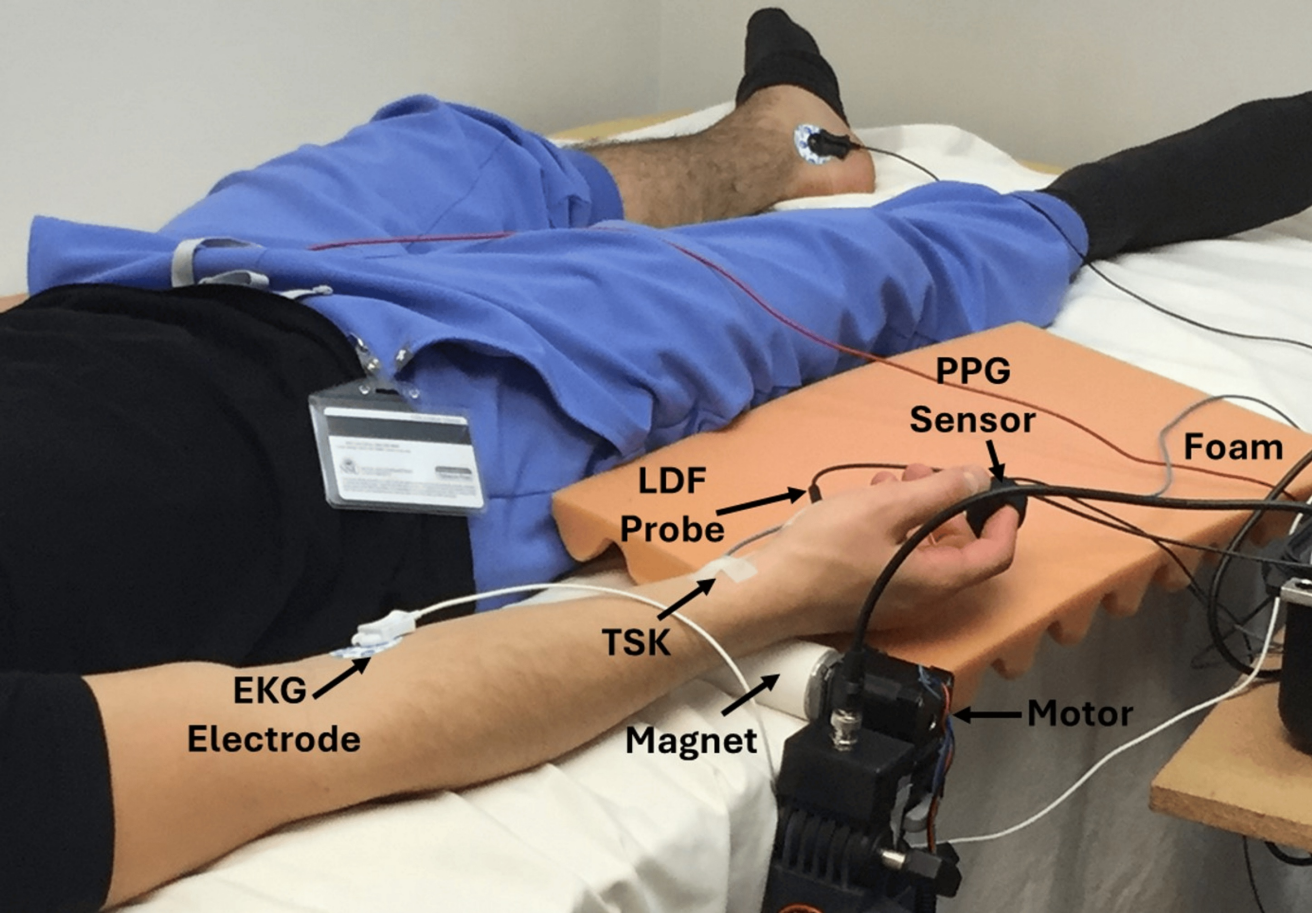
Experimental setup The arm and hand rest on a padded exam table and supportive foam material, with the body part just in contact with the protective shell encasing the rotating magnet. Skin blood perfusion is measured with a laser Doppler probe (LDF). Finger pulses are measured with a photoplethysmographic (PPG) sensor, and skin temperature is measured at the wrist.
